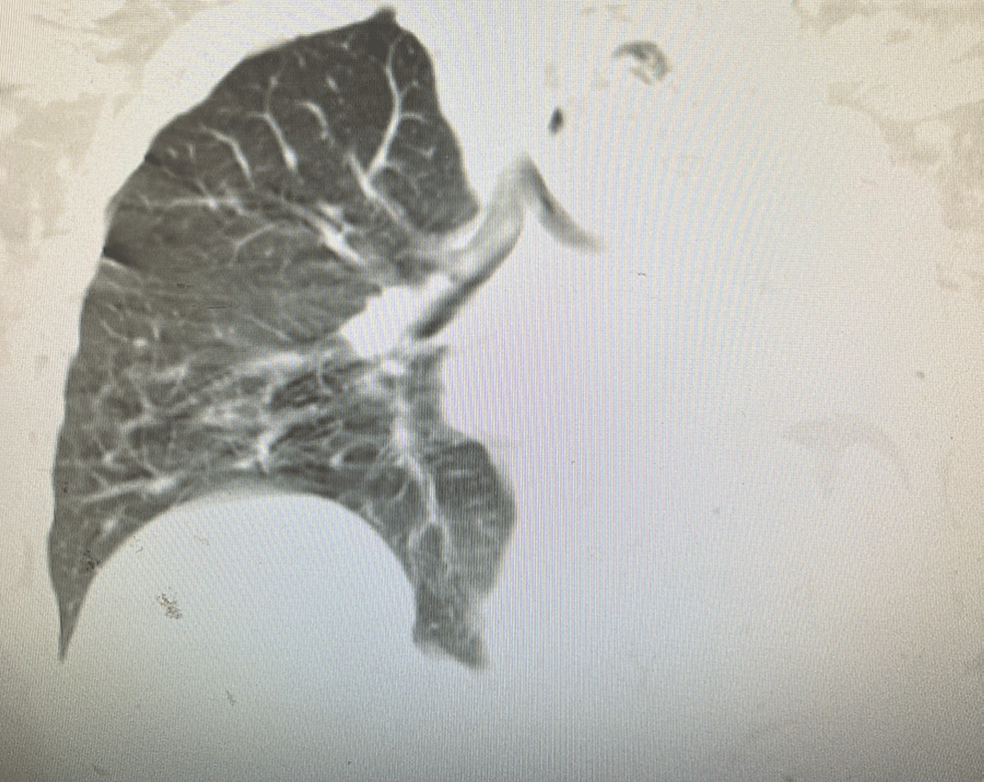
CT chest without contrast done on the day of admission
